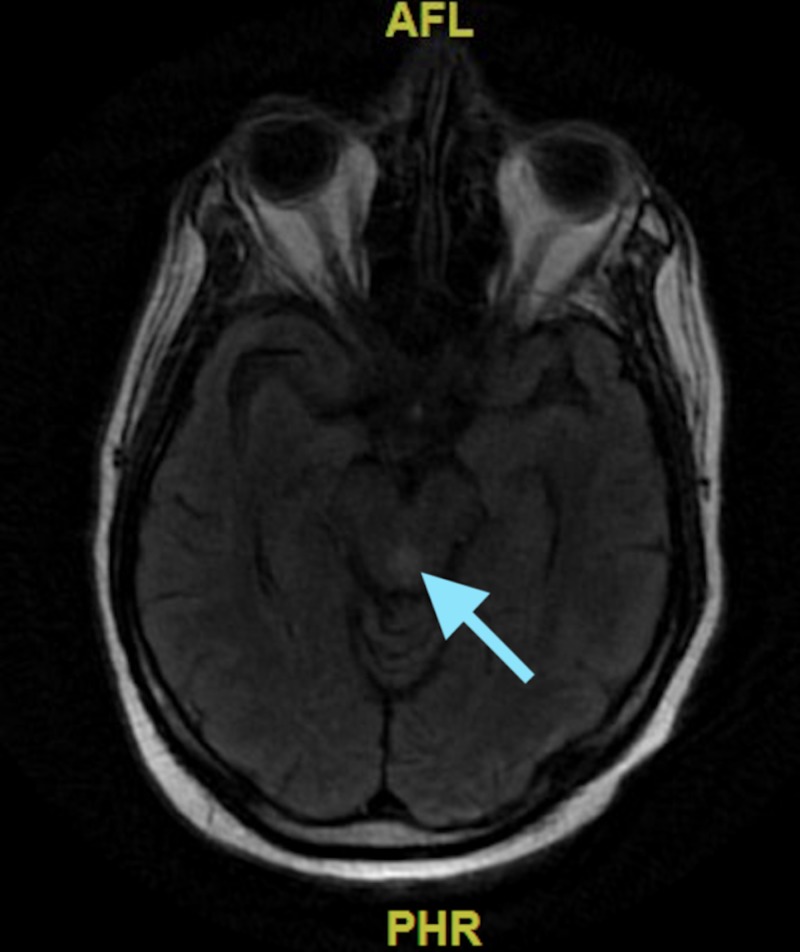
Magnetic resonance imaging of the brain (T2/FLAIR) showing hyperintensity in periaqueductal gray area depicted by the blue arrow.
